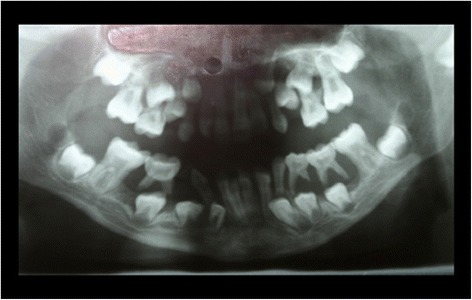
Panoramic radiograph at the age of 9 years: exhibiting signs of untreated periodontal disease.
